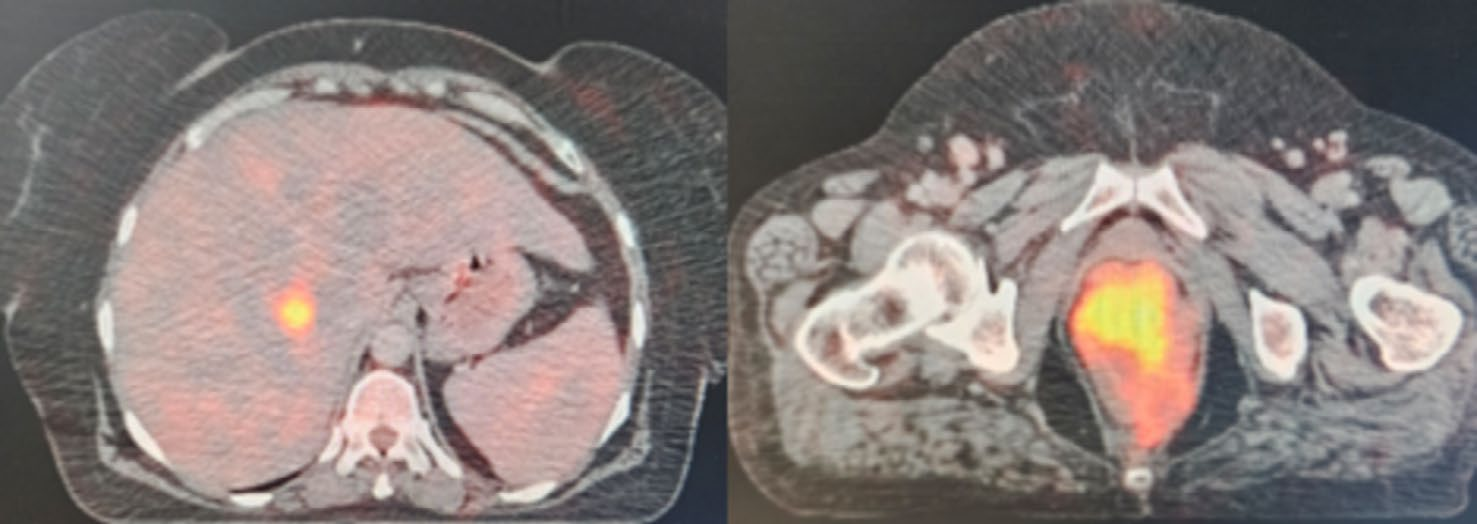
Liver metastases and the ano‐rectal growth as visualized in the baseline whole body FDG18 Positron Emission Tomography CT (PET CT).
